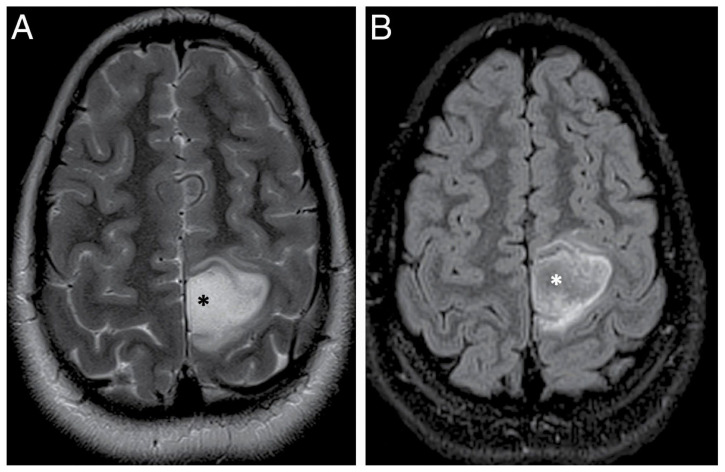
T2-FLAIR mismatch sign in an 11-year-old female patient with a diagnosis of IDH-mutant, 1p/19q non-codeleted and p53-muted anaplastic astrocytoma in the left parietal lobe. (A) Axial T2-weighted image demonstrating an ill-defined tumor in the left paracentral lobule region with almost homogenous hyperintense T2 signal at the center of the tumor (*) that is mostly hypointense on the corresponding FLAIR image (B). Reproduced with permission from: [25] © The authors 2022.
